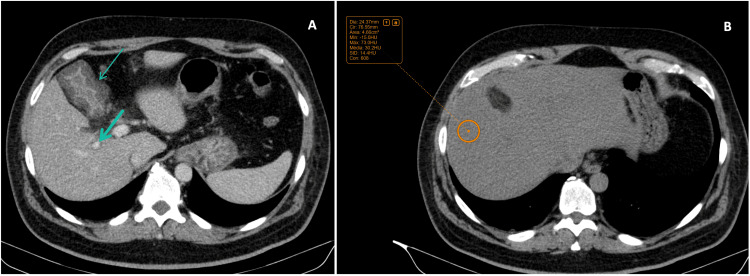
Abdominopelvic CT at first admission (A) Marked gallbladder wall thickening (thin arrow), measuring approximately 13 mm, with pericholecystic fat stranding, without luminal distension or radiopaque cholelithiasis and periportal low attenuation/halo, compatible with periportal edema (thick arrow), both non-specific findings that may be seen in acute hepatitis [11]. There was no evident intrahepatic biliary ductal dilatation. (B) Diffuse decrease in hepatic parenchymal attenuation, consistent with hepatic steatosis (mean attenuation ~30 HU).
